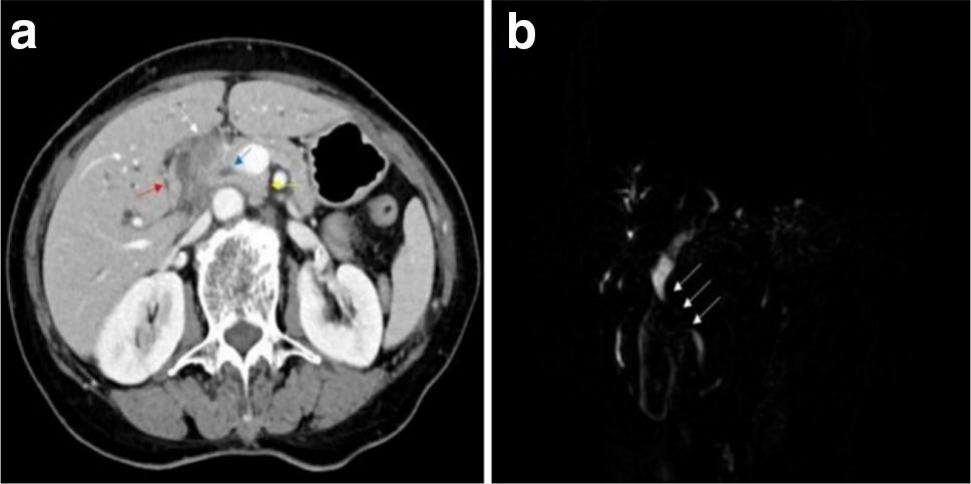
Computed tomography (a) and magnetic resonance imaging (b) showed stenosis at the common hepatic duct and the upper segment of the common bile duct (a The white arrow indicates the soft tissue shadow, the blue arrow indicates the common bile duct, the red arrow indicates the duodenum, and the yellow arrow indicates the head of the pancreas. b The white arrow indicates the stenosis.).
